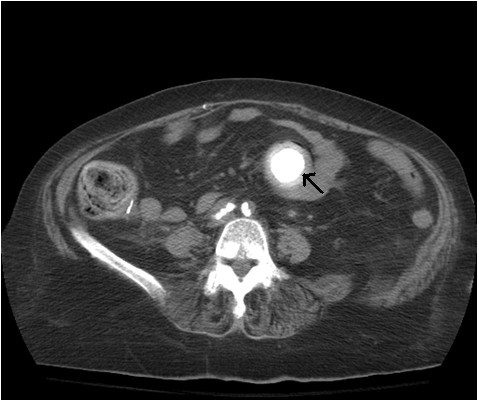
CT scan showing a gallstone that is completely obstructing the patient's duodenum.
